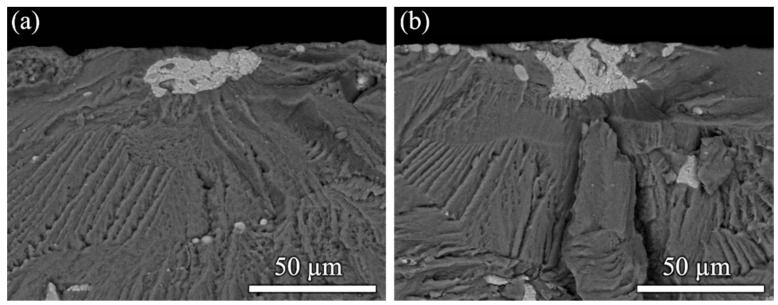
Fatigue crack initiation in 2024-T351 at a fractured secondary phase particle, probably Al_7_Cu_2_(Fe, Mn), cycling at stress amplitude 145 MPa (a) in ultrasonic test (1.8 × 10^5^ cycles to failure) and (b) in servo-hydraulic test (9.1 × 10^4^ cycles to failure).
